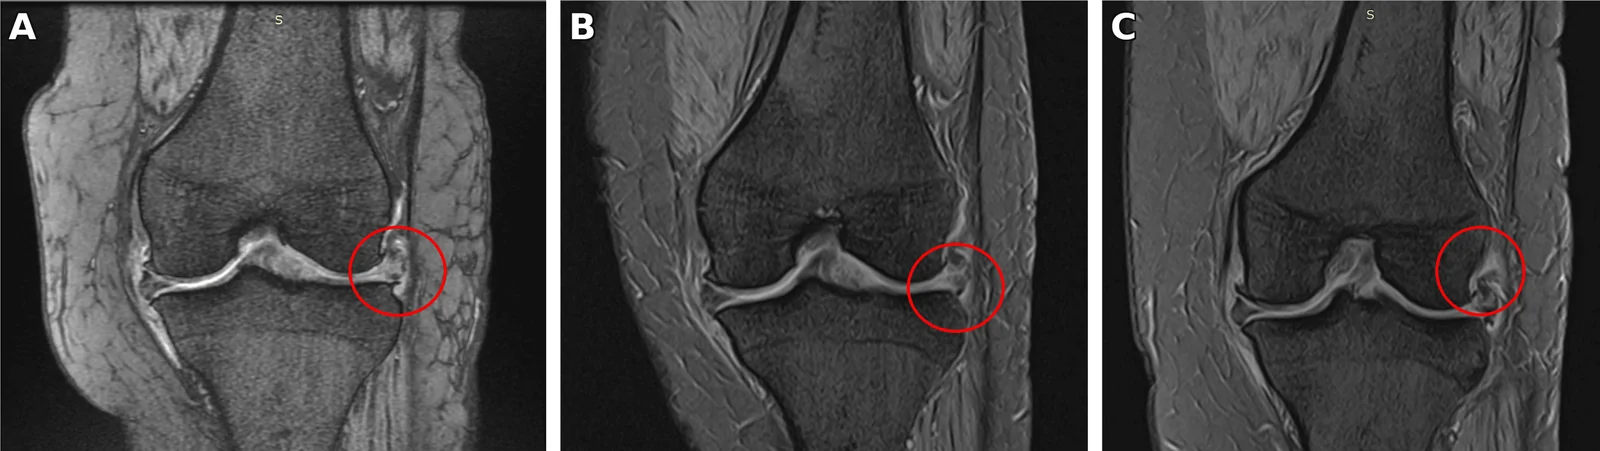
Serial coronal T2*-weighted MRI of the left knee before and after intra-articular ADMSC injection Serial coronal T2*-weighted MRI of the left knee. (A) Pretreatment (Day -52); (B) Day 78; (C) Day 407. Red circles indicate the lateral meniscal lesion, which improved in signal and morphology over time. Panel A was acquired on a different scanner. ADMSC: adipose-derived mesenchymal stem cell
